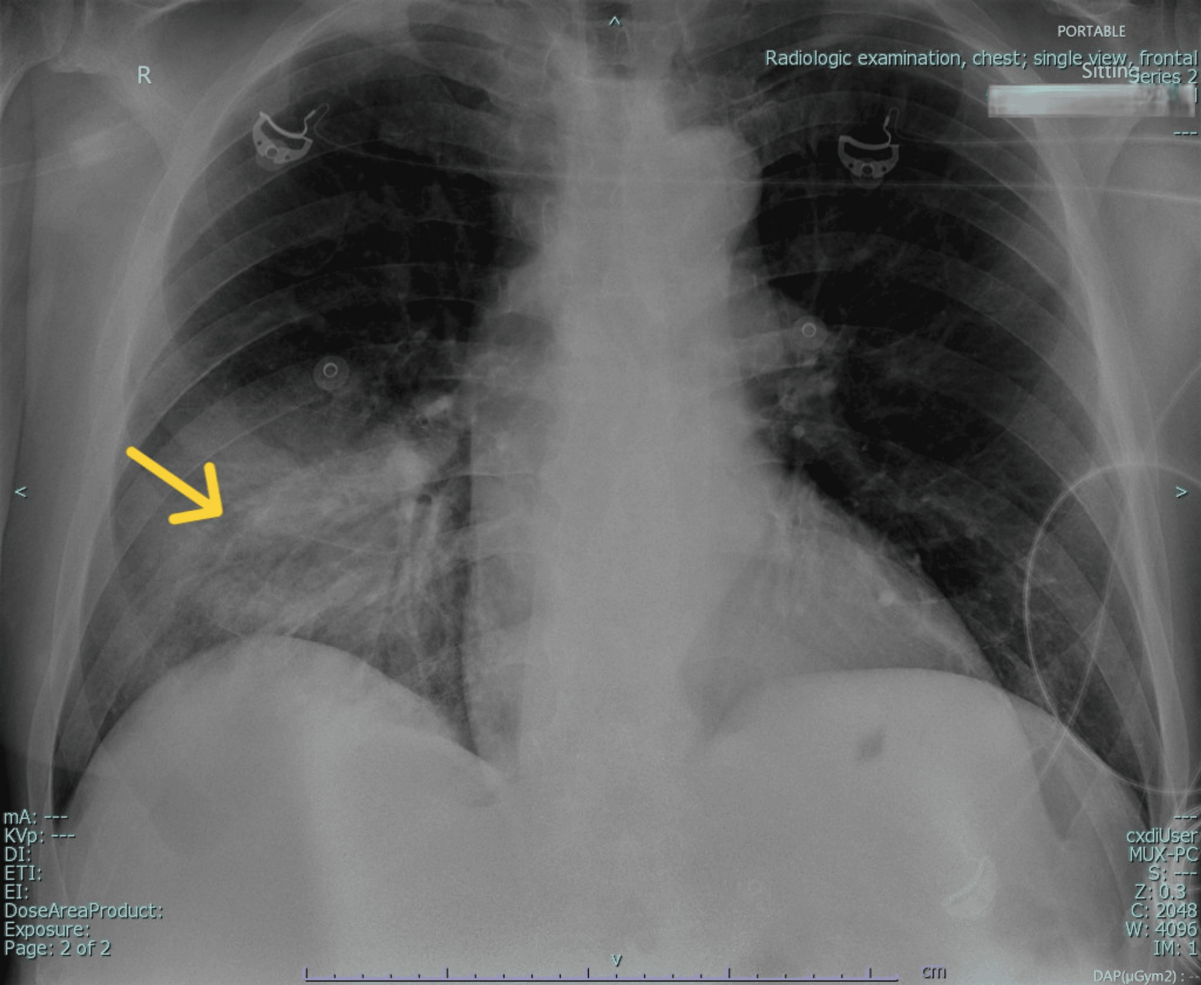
Chest x-ray (portable, anteroposterior view) showing a focal heterogeneous opacity in the right lower lobe, suggestive of focal pneumonia (yellow arrow).
